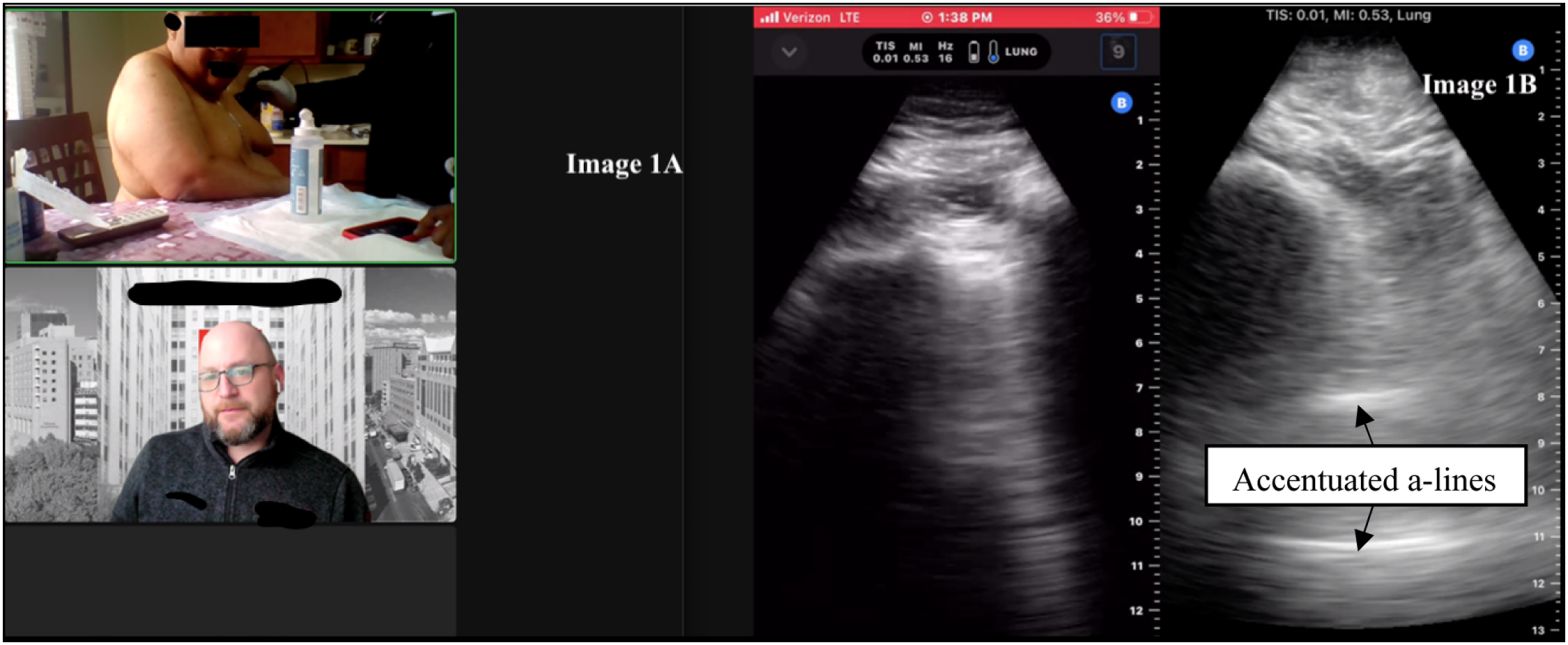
(A) Example of a community tele-paramedic visit with video conference and screen sharing using mobile ultrasound. The patient is seen at the top, and the emergency physician is seen on the bottom screen. (B) The case patient’s b-mode lung point-of-care ultrasound, showing accentuated a-lines, loss of b-lines, and in real-time video without lung sliding (not pictured here).
